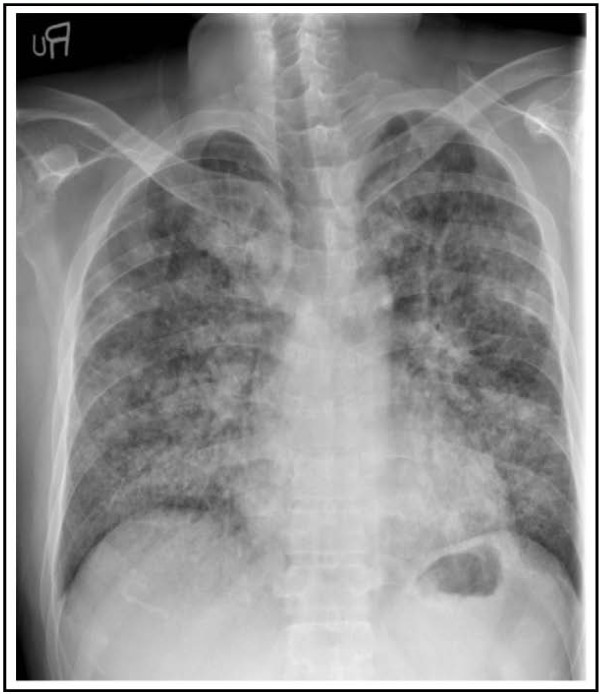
Patch density in chest X-ray was progressed, suggestive of acute respiratory distress syndrome.
